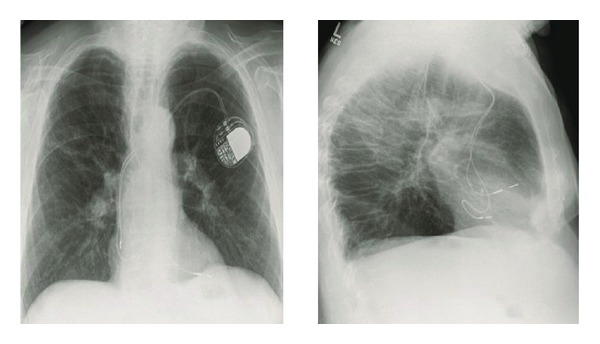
Chest radiograph PA and lateral projection showing the ventricular lead with an abnormal configuration.
